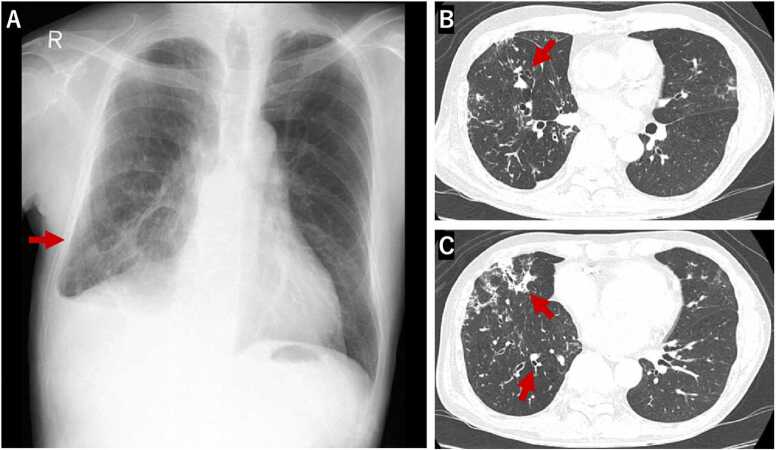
Chest radiograph and computed tomography (CT) scan before diagnosis. (A) The chest radiograph obtained in May 2020, shows ground-glass opacity in the right lower lung field. (B, C) Chest CT scan reveals centrilobular nodules and bronchial wall thickening in the right middle lobe, which raised concern for lower respiratory tract infection.
